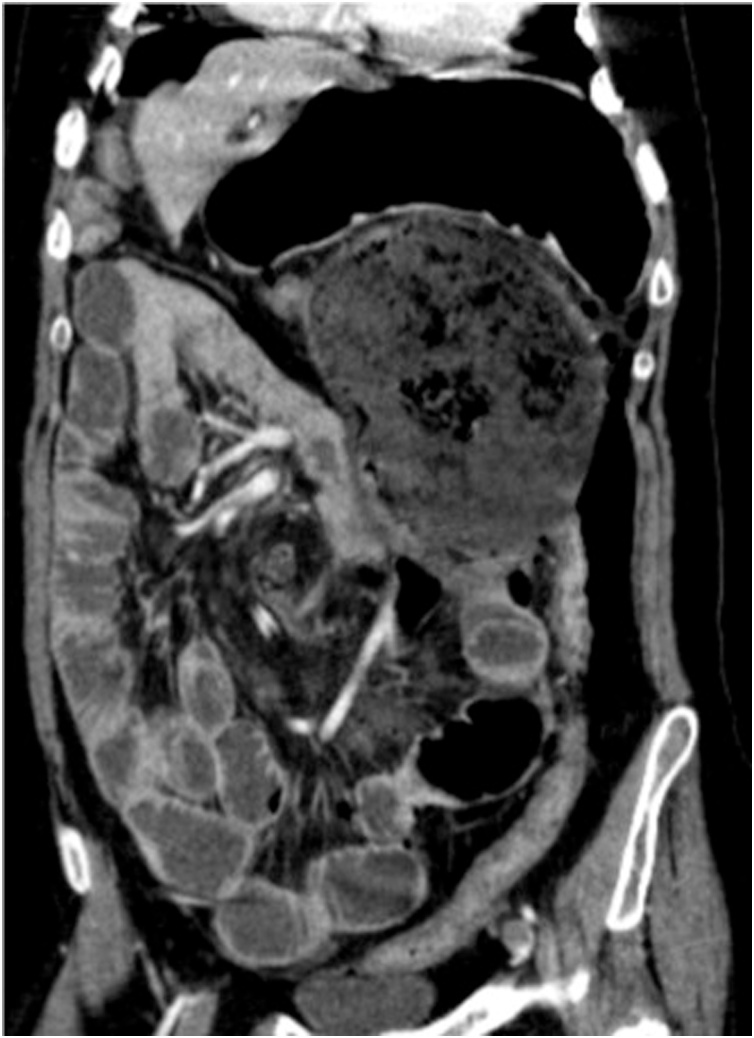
Abdominal CT scan evidencing intestinal malrotation with high intestinal obstruction due to compression of the stomach by the cecum.
